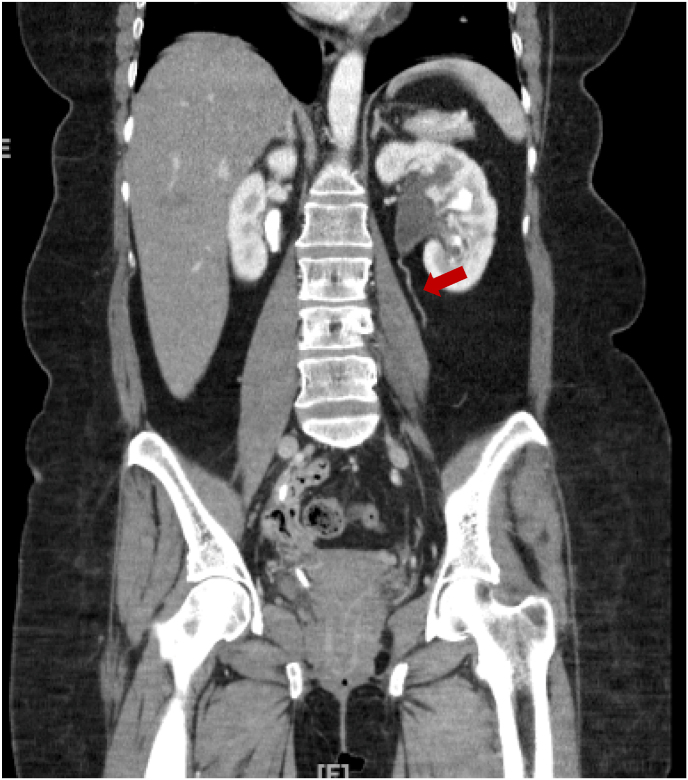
Coronal view of CT Abdomen/Pelvis with contrast taken a month prior. *Red arrow* points to small filling defects in the distal left ureter, that was due to fungus balls found intraoperatively. (For interpretation of the references to colour in this figure legend, the reader is referred to the Web version of this article.)
